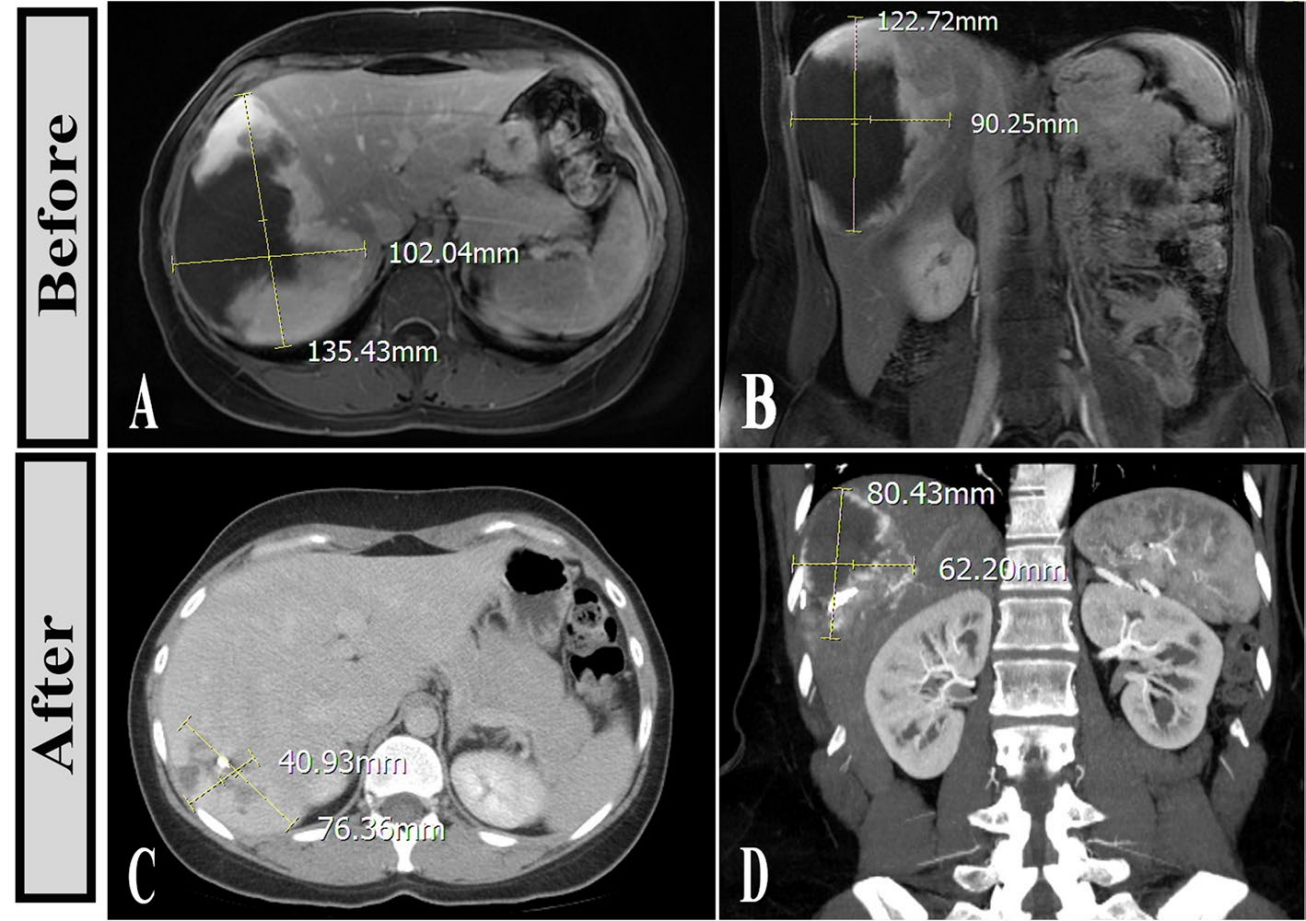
A, B MRI of a 37-year-old woman with vague abdominal pain, showing a giant HVM in the right liver lobe with typical enhancement. C, D Nine months after treatment with bleomycin and lipiodol, significant reduction in size is notable. Small clumps of lipiodol are seen within the lesion on control CT scan as dense spots
